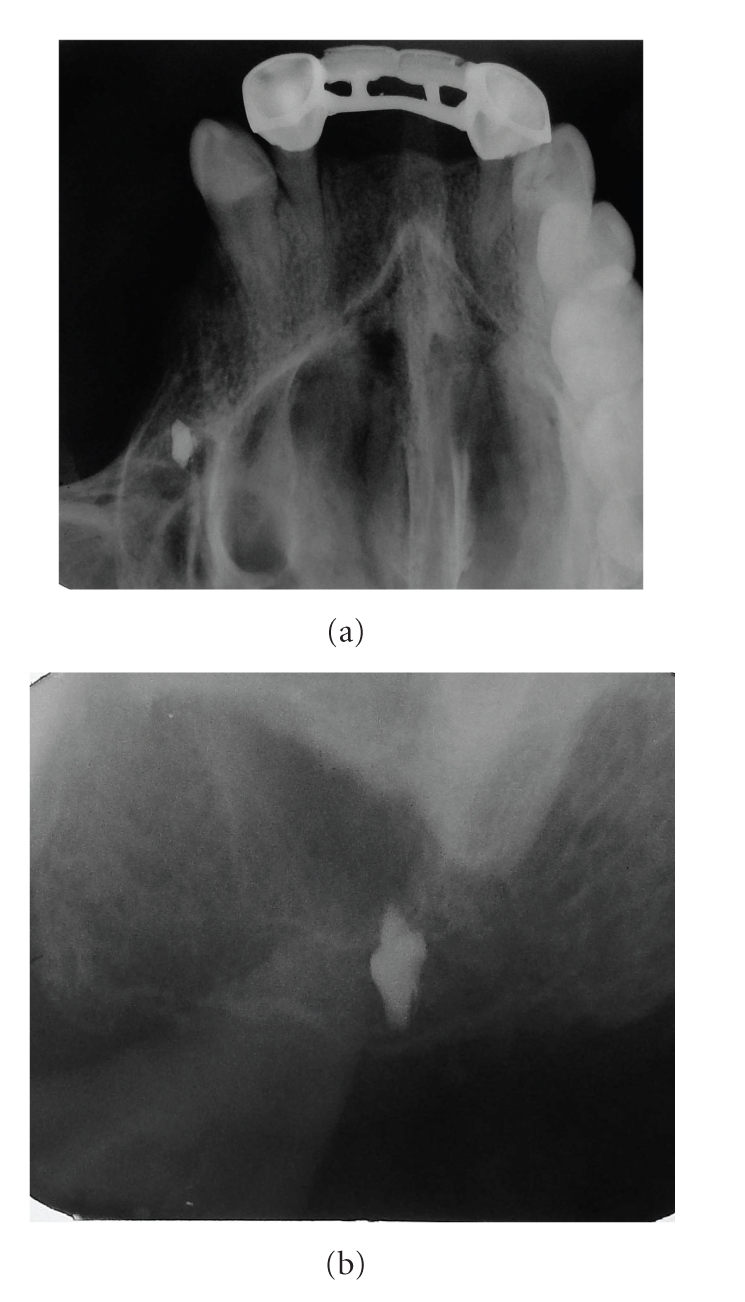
(a) Maxillary occlusal X-ray. (b) Periapical X-ray. Notice the closure of the foreign body to the right maxillary sinus, and also a nonhealed 3 alveolus.
